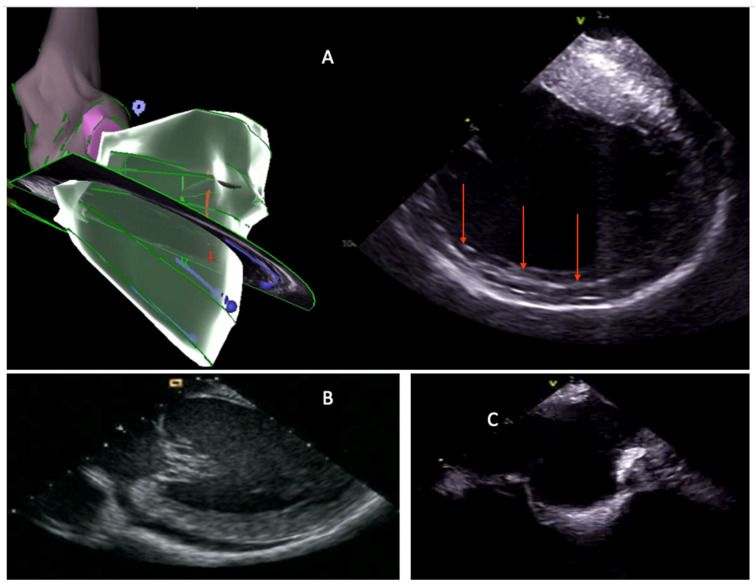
Examples of applications of ICE in VT ablation: (A) 3D reconstruction of the left ventricle obtained with the ICE probe positioned in the right ventricle, showing a subendocardial scar area on the lateral wall (red arrows); (B) intraprocedural detection of pericardial effusion; (C) identification of a large aortic plaque at the sino-tubular junction, which prompted the operator to avoid the transaortic approach and proceed exclusively via the transseptal route. ICE, intracardiac echocardiography; VT, ventricular tachycardia.
